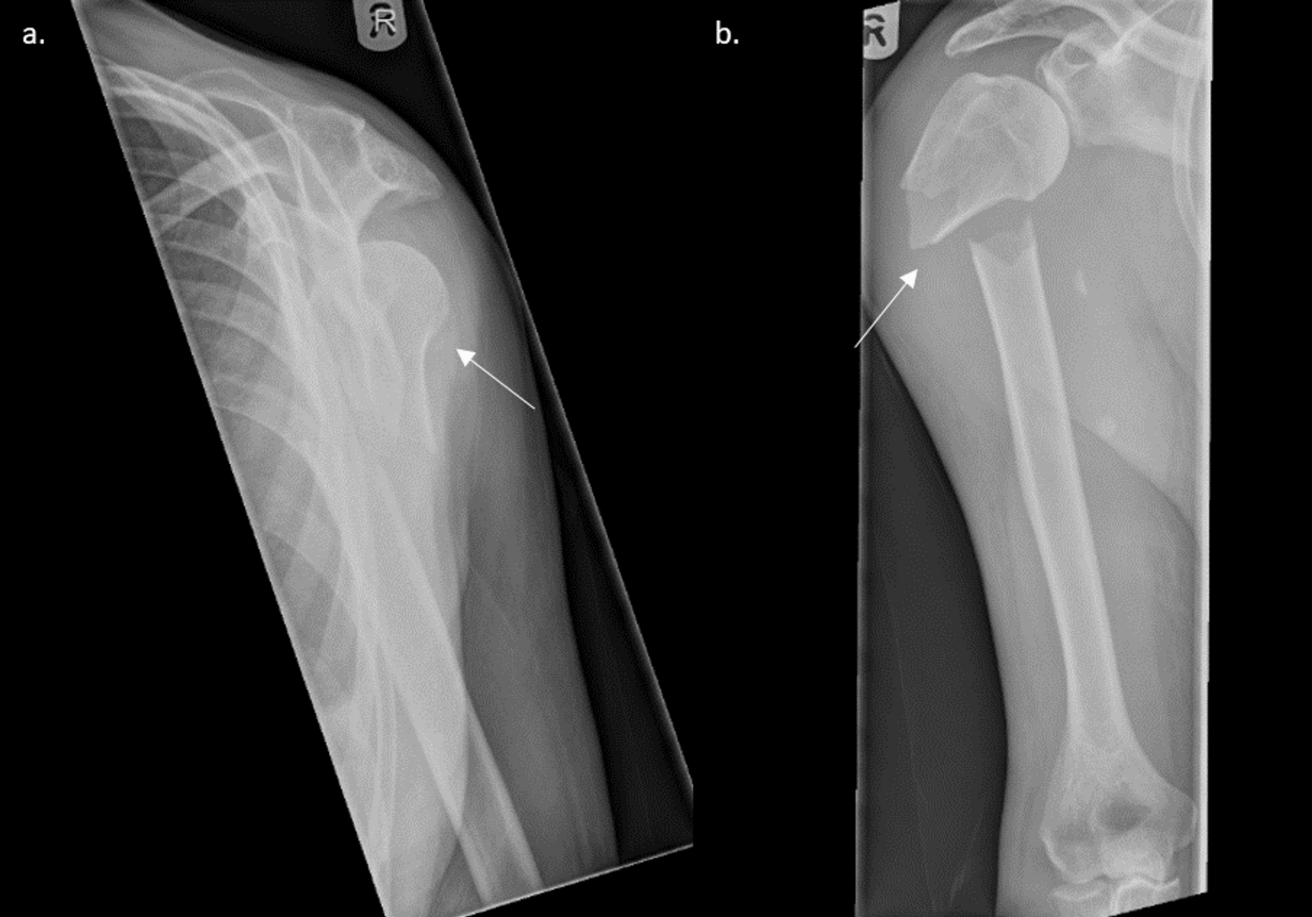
Preoperative anteroposterior radiographs of the right shoulder demonstrating a displaced proximal humeral fracture: (a) full-length shoulder view and (b) magnified view of the proximal humerus The white arrows indicate the fracture.
